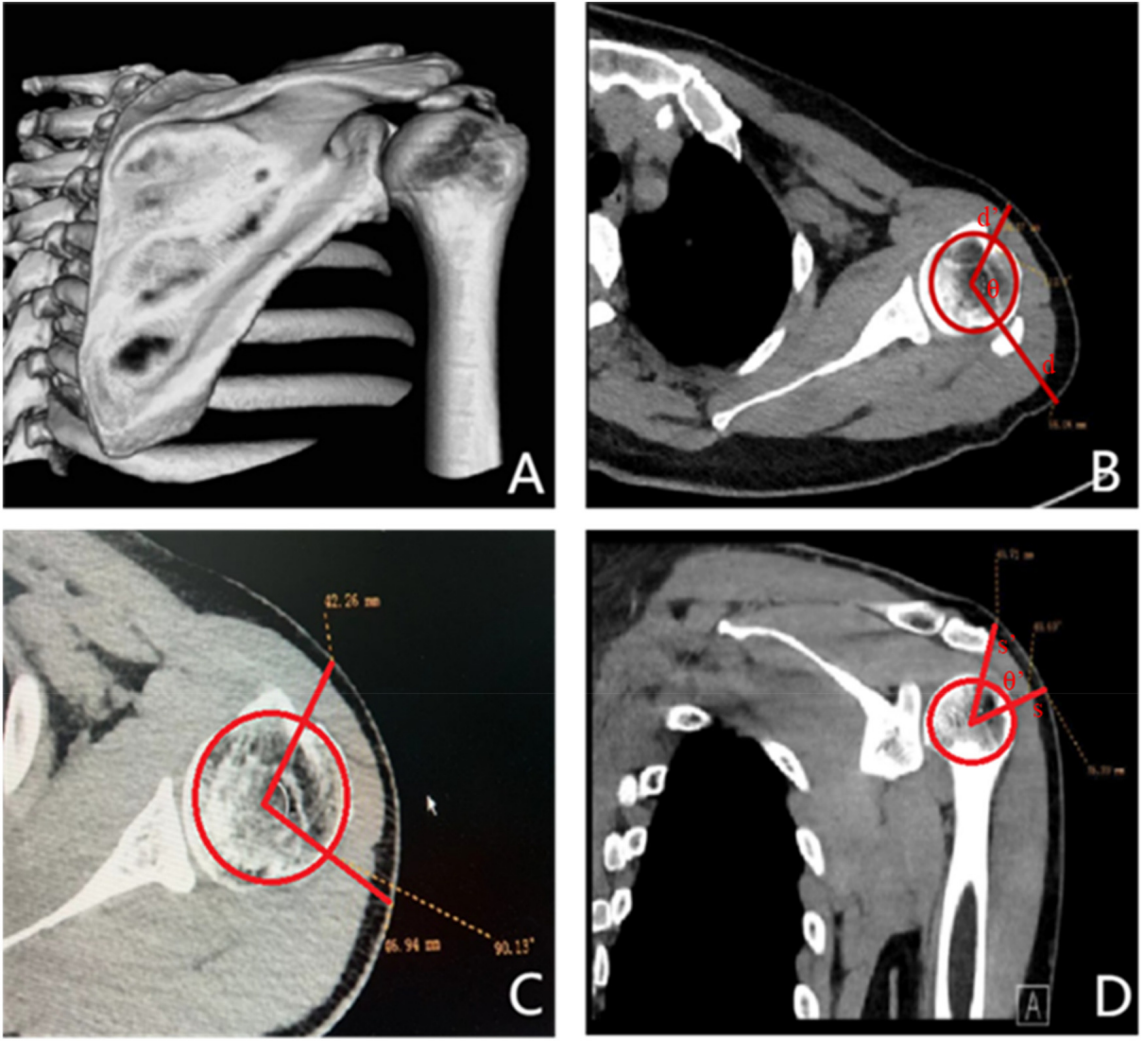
(A) This figure is a 3D CT image of the shoulder joint, showing calcium deposits within the shoulder joint. (B,C): These figures show the cross-sectional CT of the shoulder joint and the measurements of d, d ‘and *θ*. (D): This figure shows the coronal CT of the shoulder joint, measuring the value of s, s’, θ ’.
